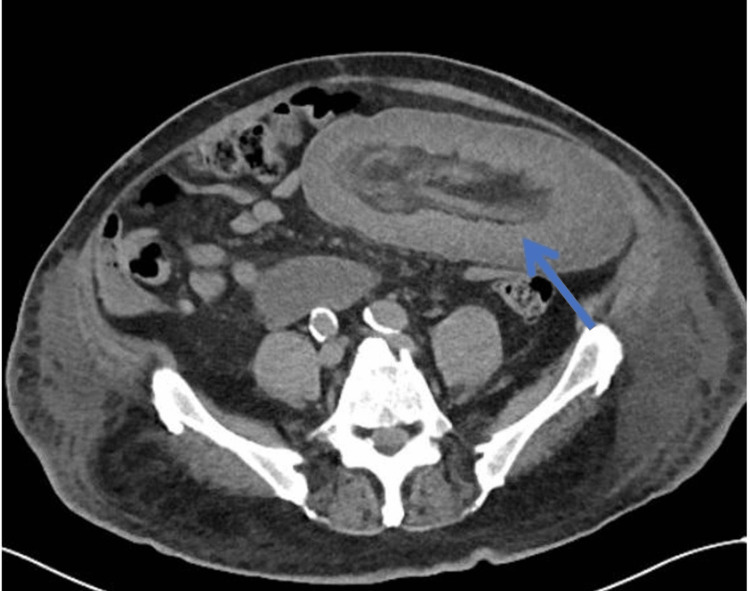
CT of the abdomen showing a large long segment likely jejunojejunal intussusception resulting in small bowel obstruction with edematous changes of the involved bowel loops (arrow).
